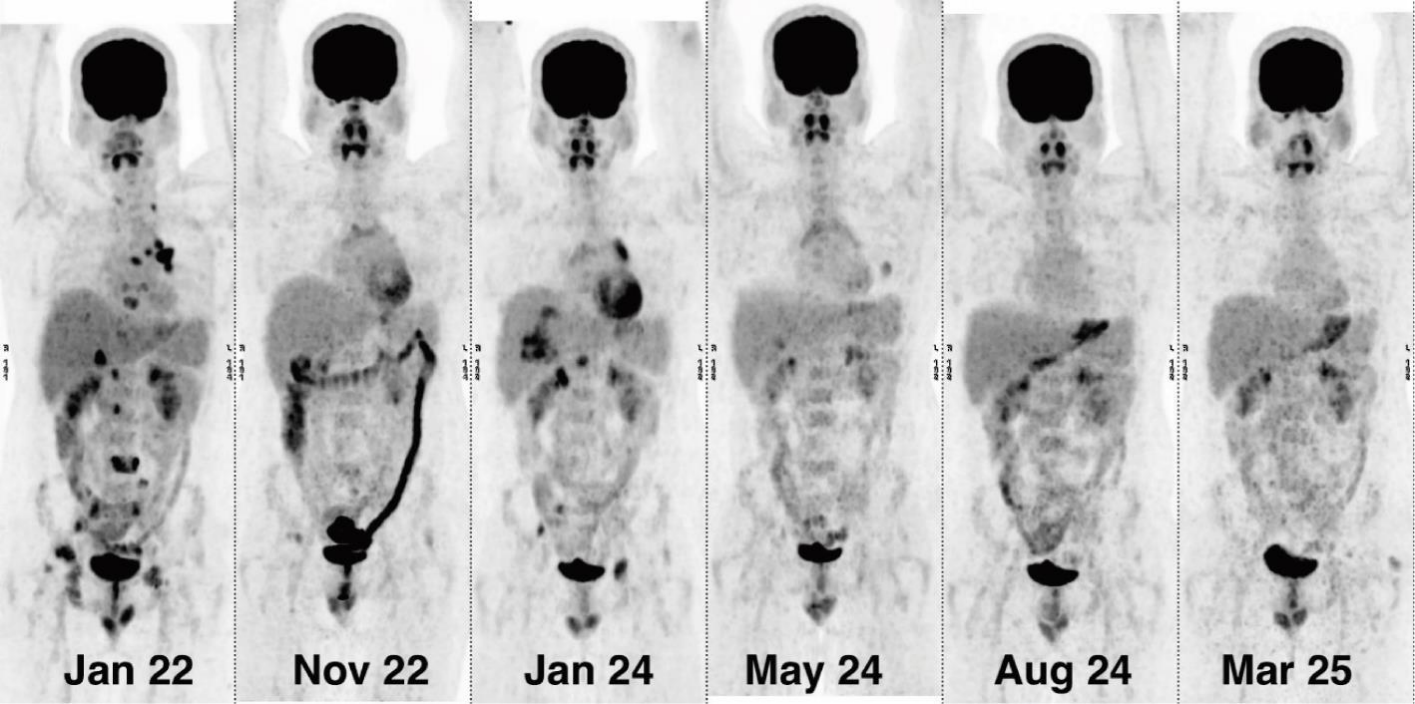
Serial scout images of FDG PET‐CT showing progressive liver, nodes, and bony lesions on January 24, which responded completely in the scan of May 24 after starting brigatinib, and the response was maintained in scans of August 24 and March 25.
